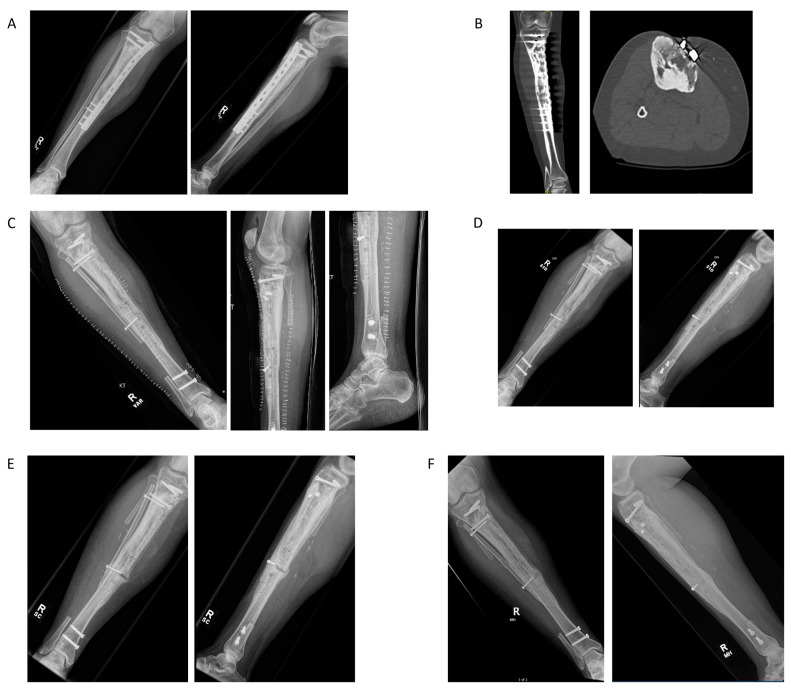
(A) Pre-operative AP and lateral radiographs demonstrating prior segmental resection of the proximal right tibia with allograft secured with a medial plate and multiple screws. (B) Pre-operative coronal and axial computed tomography (CT) images demonstrating potential hardware failure and concern for allograft fracture. (C) Immediate post-operative AP and lateral radiographs showing carbon fiber intramedullary rod in place and transposed vascularized fibular autograft secured with two screws. Note is made of one broken screw fragment from her prior operation that was retained in the proximal tibia. (D) AP and lateral radiographs 4-months post-operatively demonstrating a new unicortical allograft fracture after mechanical fall. (E) AP and lateral radiographs 8-months post-operatively bridging callus at the site of the mid-tibial unicortical allograft fracture. (F) AP and lateral radiographs 2-years post-operatively showing healed mid-tibial unicortical allograft fracture.
